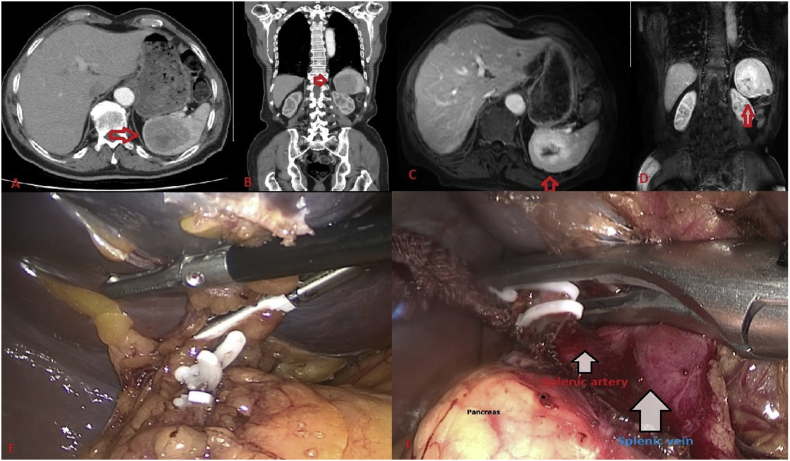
Abdominal CT (A&B) and Abdominal MR (C&D) show SANT with red arrows. (For interpretation of the references to color in this figure legend, the reader is referred to the Web version of this article.)
